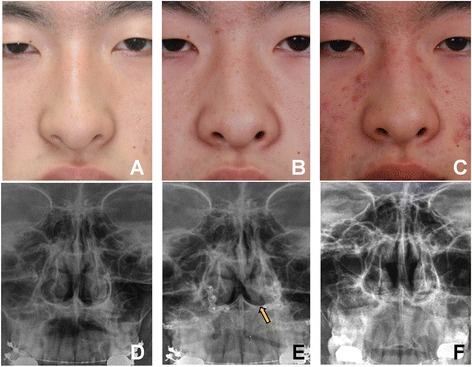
A Facial photographs and cephalo PA radiograph of the patient (case 1) before Le Fort I osteotomy (a, d), immediately after the maxillary osteotomy that showed the deviation of the septum to the left side (b, e). The *arrow* indicates the caudal part of the septal cartilage (e). After the surgical correction of nasal septum deviation (c, f)
